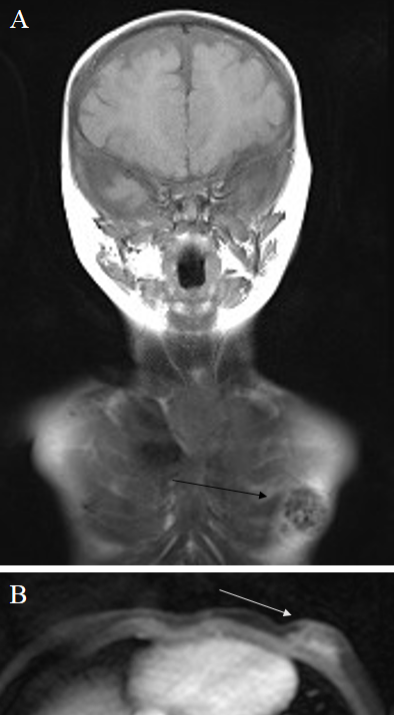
Magnetic resonance images of the NF1-affected child A) On T1-weighted images (coronal plane), an inhomogeneous roundish space-occupying lesion is visible in the left breast region. B) The same region is hyperintense on T2-weighted images (axial plane, cropped image).
